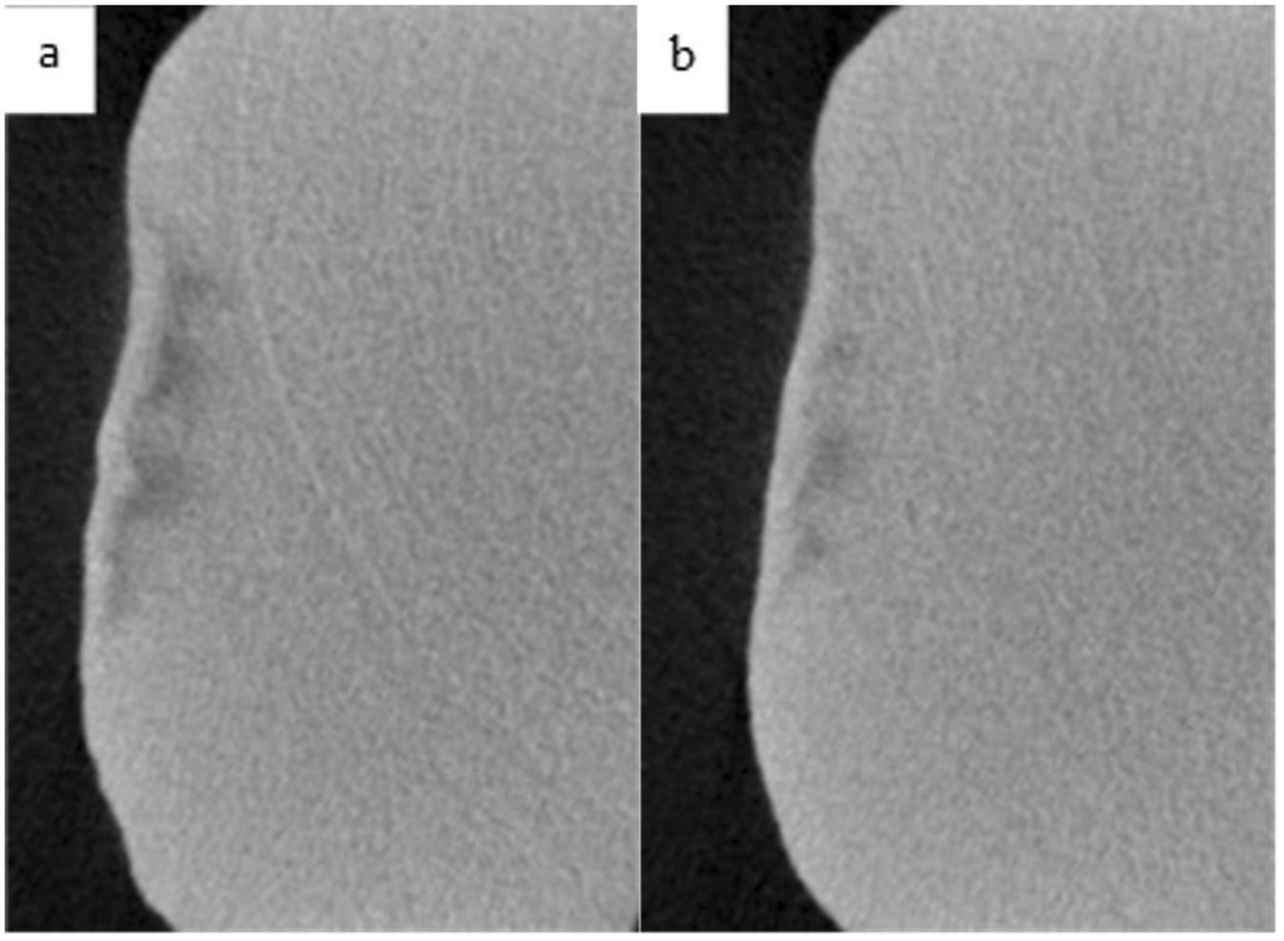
Remineralization of etched enamel MIH lesions with 20% hydroxyapatite toothpaste. Representative µ-CT images of etched enamel MIH lesions, before (a) and after (b) in-situ remineralization.
